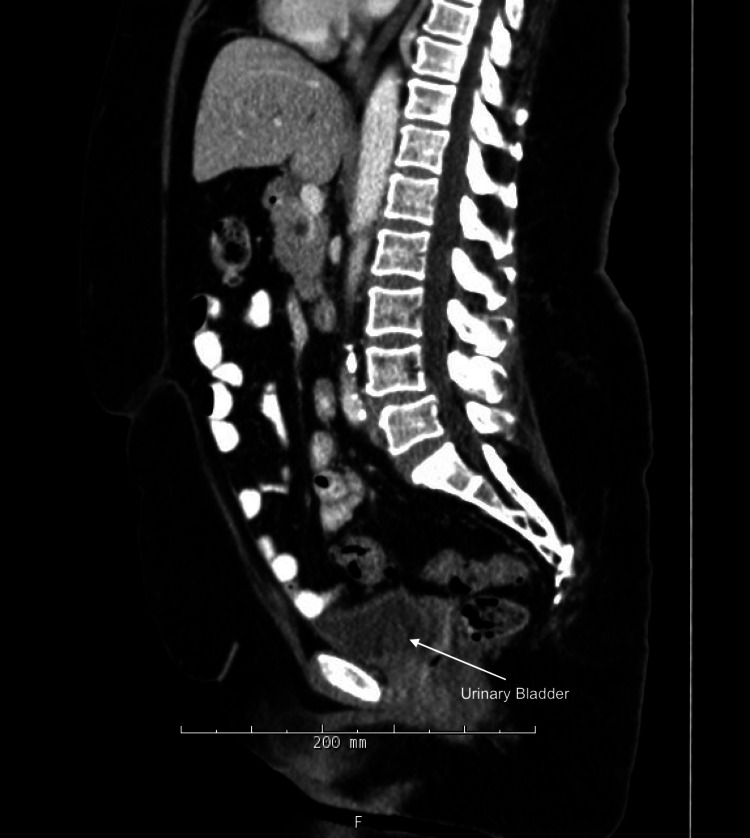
Postoperative sagittal CT of the abdomen and pelvis demonstrating no evidence of disease recurrence. CT: computed tomography
